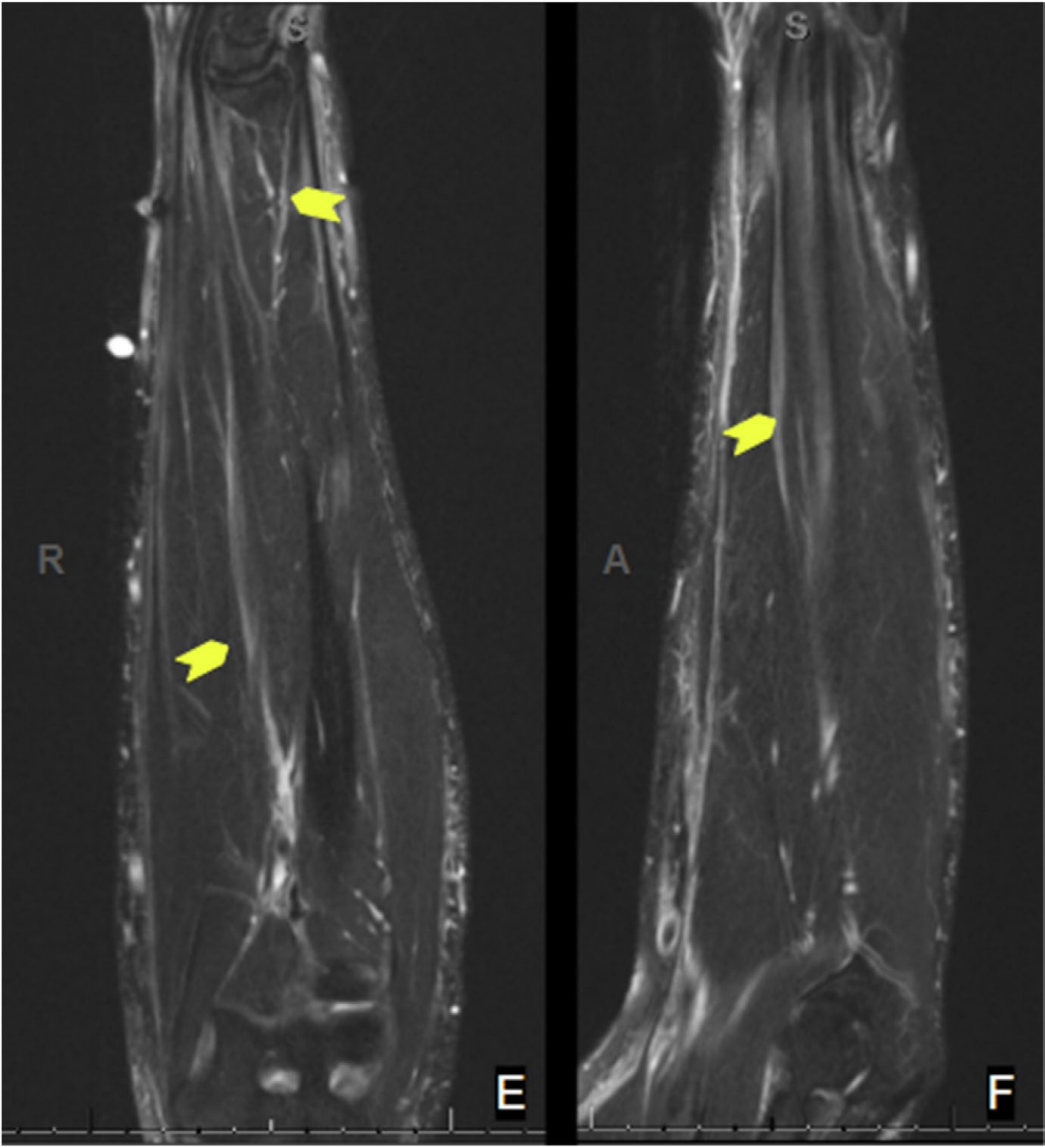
Coronal (E) and sagittal (F) left forearm axial T2‐weighted MRI images demonstrating increased signal in the subcutaneous fascia.
